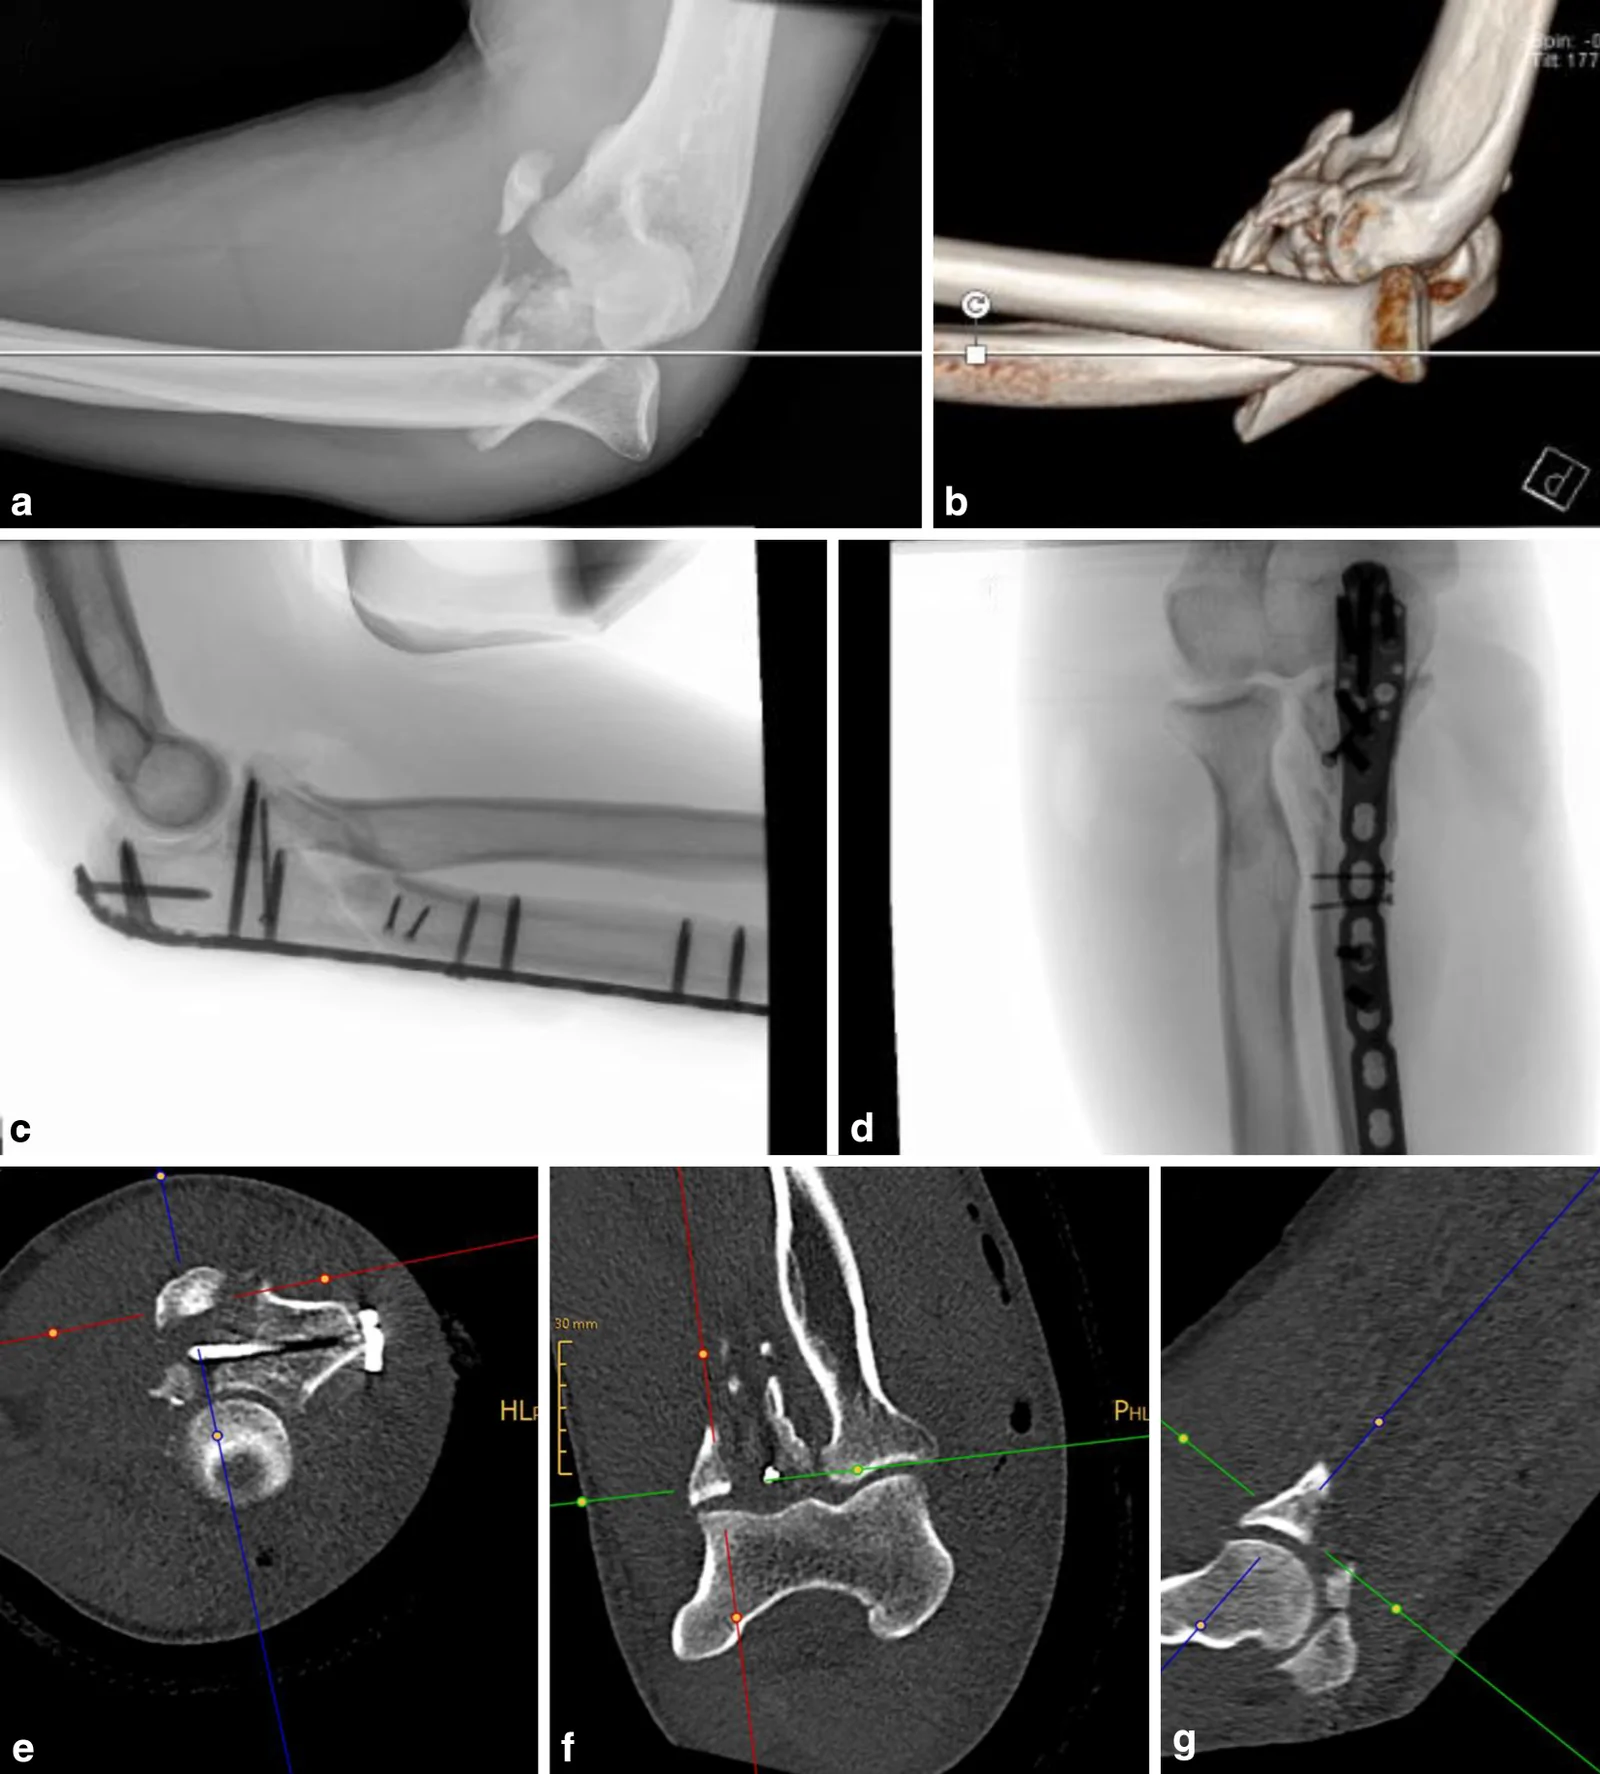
A comminuted Monteggia fracture (a, b) underwent proximal ulna fixation (c, d). Postoperative computed tomography demonstrated a malreduced coronoid (e–g)
